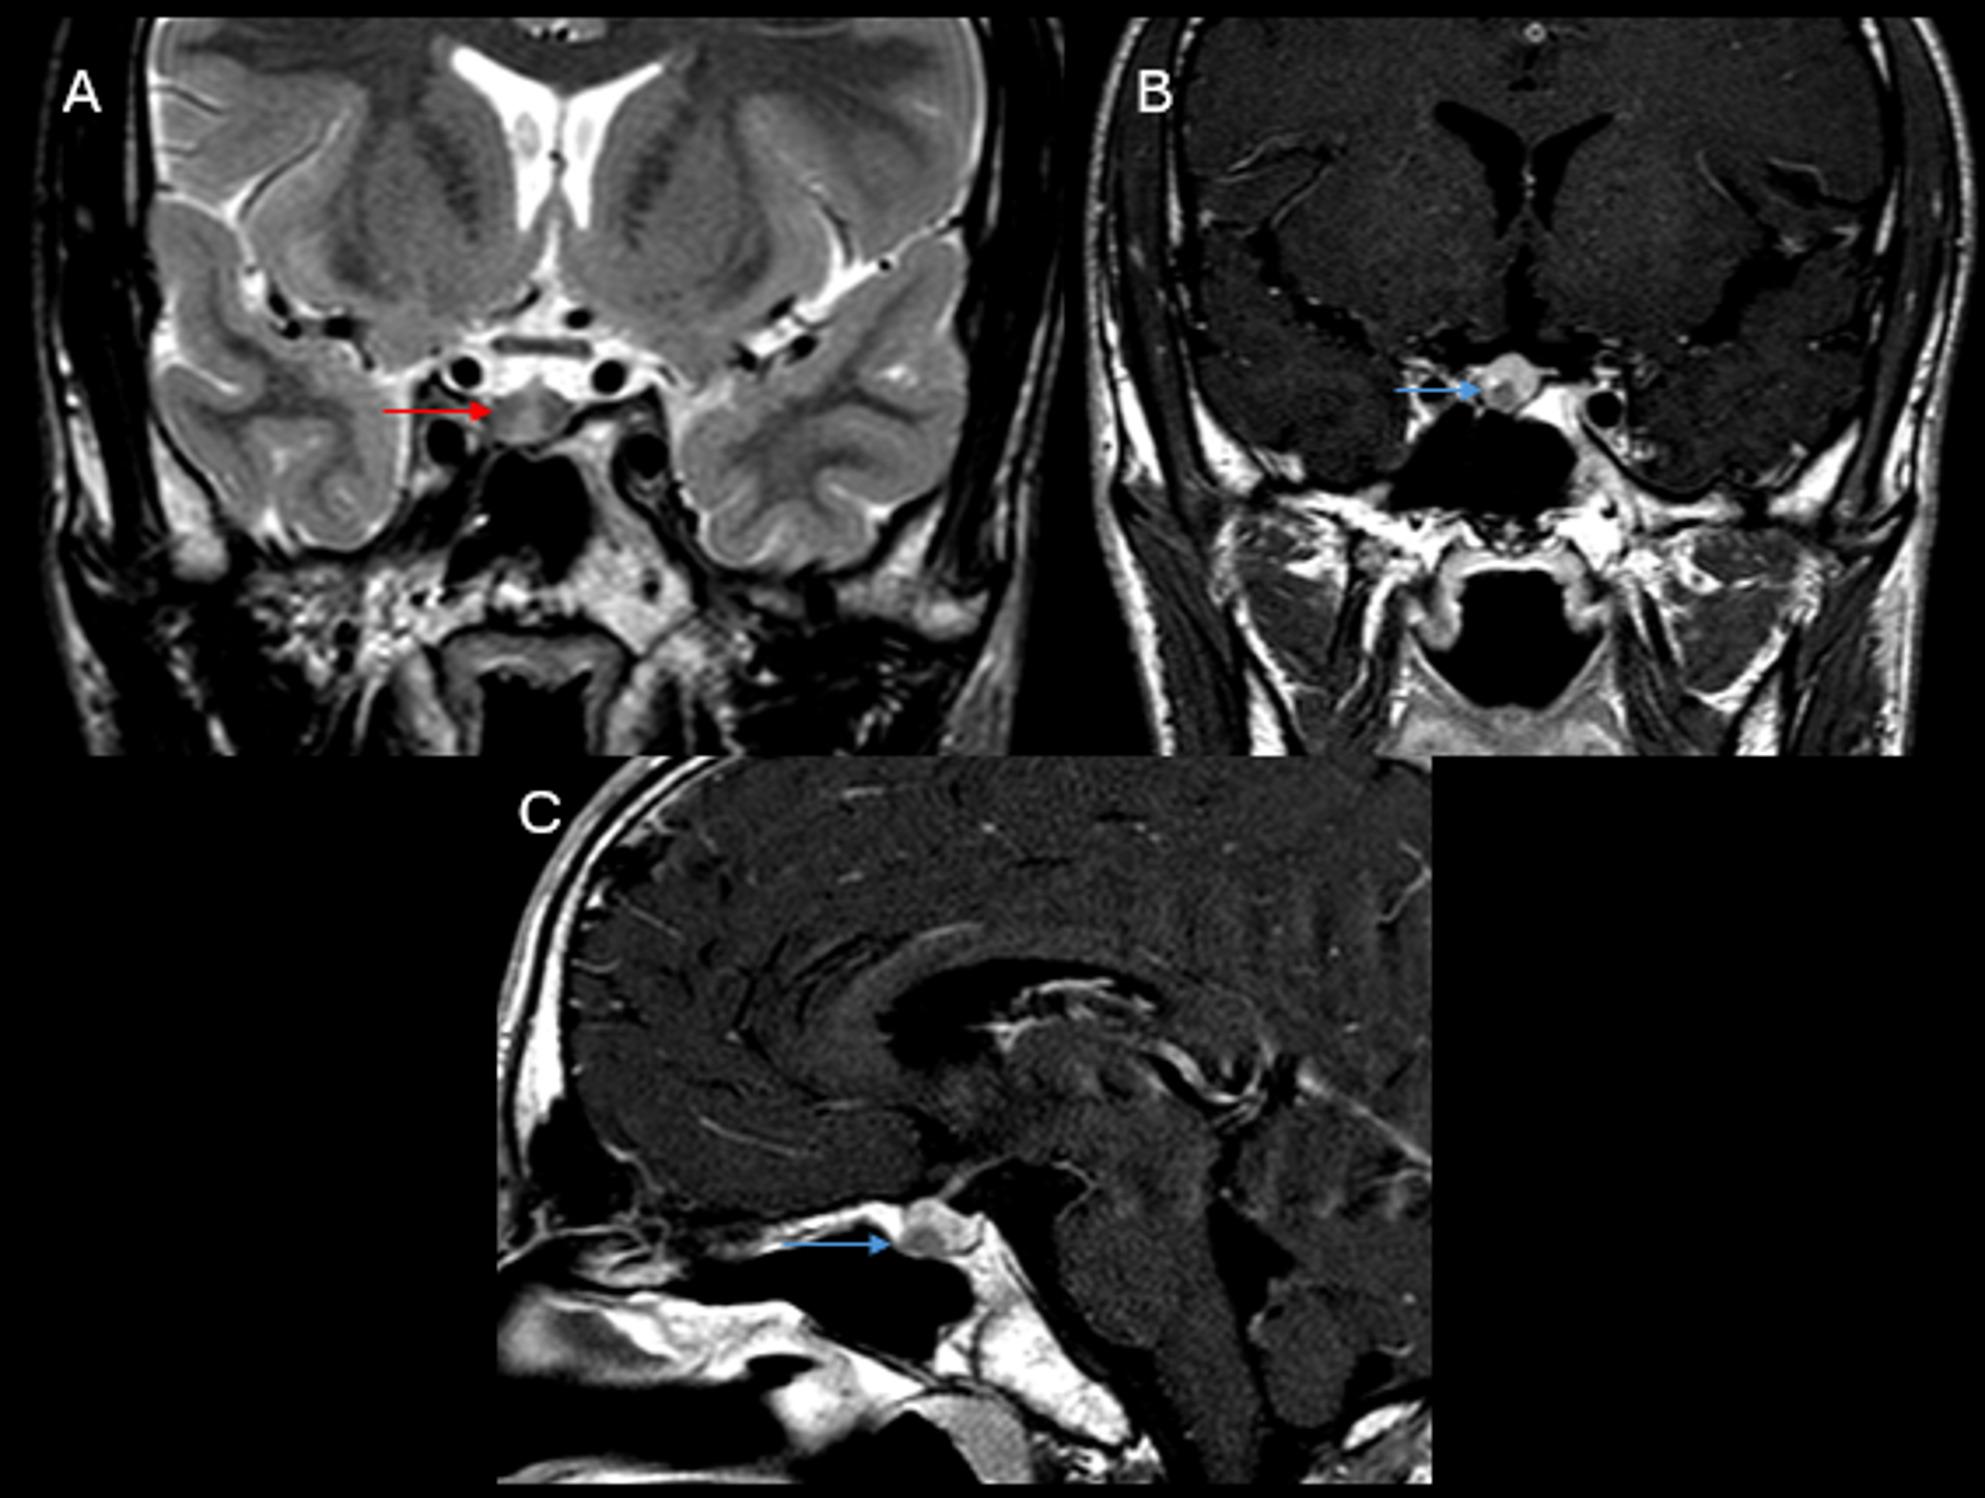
Patient with multiple endocrine neoplasia 1 with recurrent microadenoma. (A) Coronal T2-weighted image (WI), and post-contrast coronal (B) and sagittal (C) T1WI demonstrate a small well-circumscribed hypoenhancing nodule in the inferior adenohypophysis near the floor of the sella turcica (blue arrows in B and C). The lesion is hyperintense on T2 WI (red arrow in A), consistent with small pituitary microadenoma. There is no involvement of the cavernous sinuses or carotid arteries
